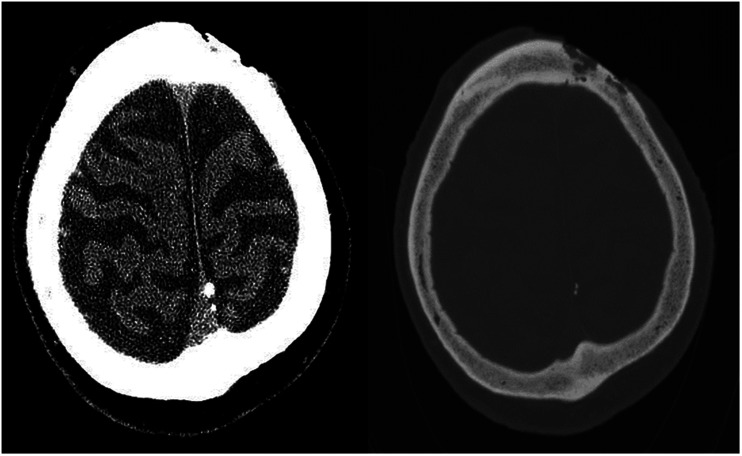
Non-enhanced CT head showing a localised skin defect in the left high frontal region with deep underlying bone erosion involving the outer and inner tables of the underlying calvarium. The permeative bone changes are multifocal and extend over approximately 3 cm diameter. No extension beyond the inner table is seen, with no evidence of involvement of the dura or brain parenchyma.
